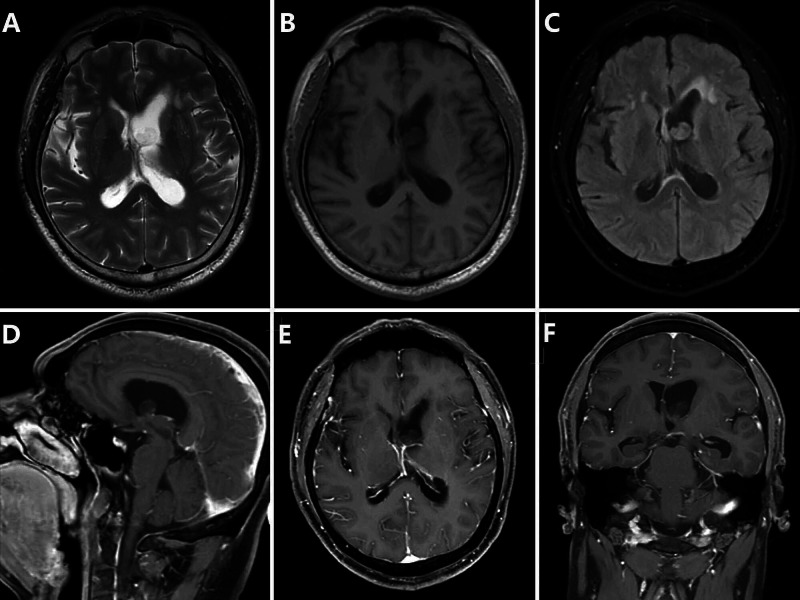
MRI of the patient. T2 and T2-FLAIR axial images demonstrating a clearly hyperintense lesion (A, C). T1-weighted axial image demonstrating isointensity relative to gray matter (B). T1-weighted axial image with gadolinium administration that did not reveal any enhancement (D, E, F). FLAIR: fluid-attenuated inversion recovery.
